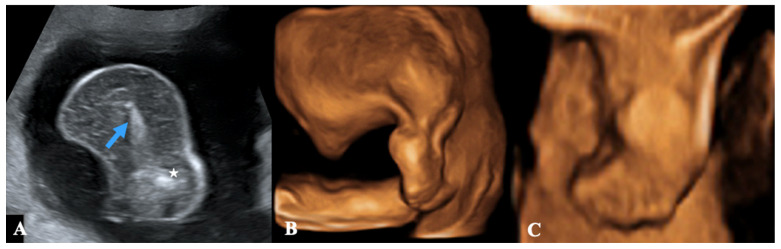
A transabdominal ultrasound in a 22-week pregnant patient. (A) A 2D ultrasonography (longitudinal view) showing an amorph mass with a lower limb consisting of femur (blue arrow pointing to part of the femur) and sketches of tibia and fibula (star). (B) A 3D ultrasonography (lateral view) confirming the presence of thigh, leg, and foot. (C) A 3D picture (frontal view) showing in detail the foot with five toes.
